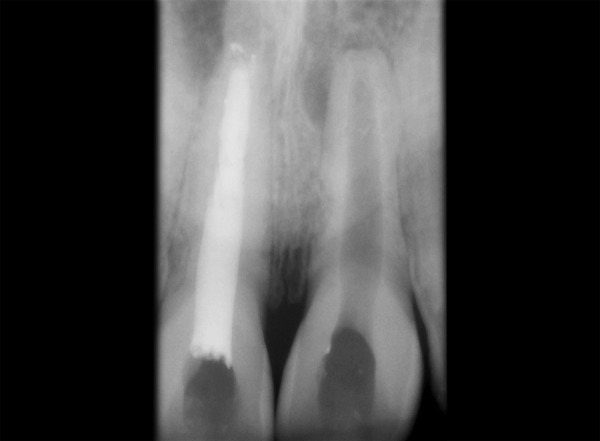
Radiographic appearance at 6 months
